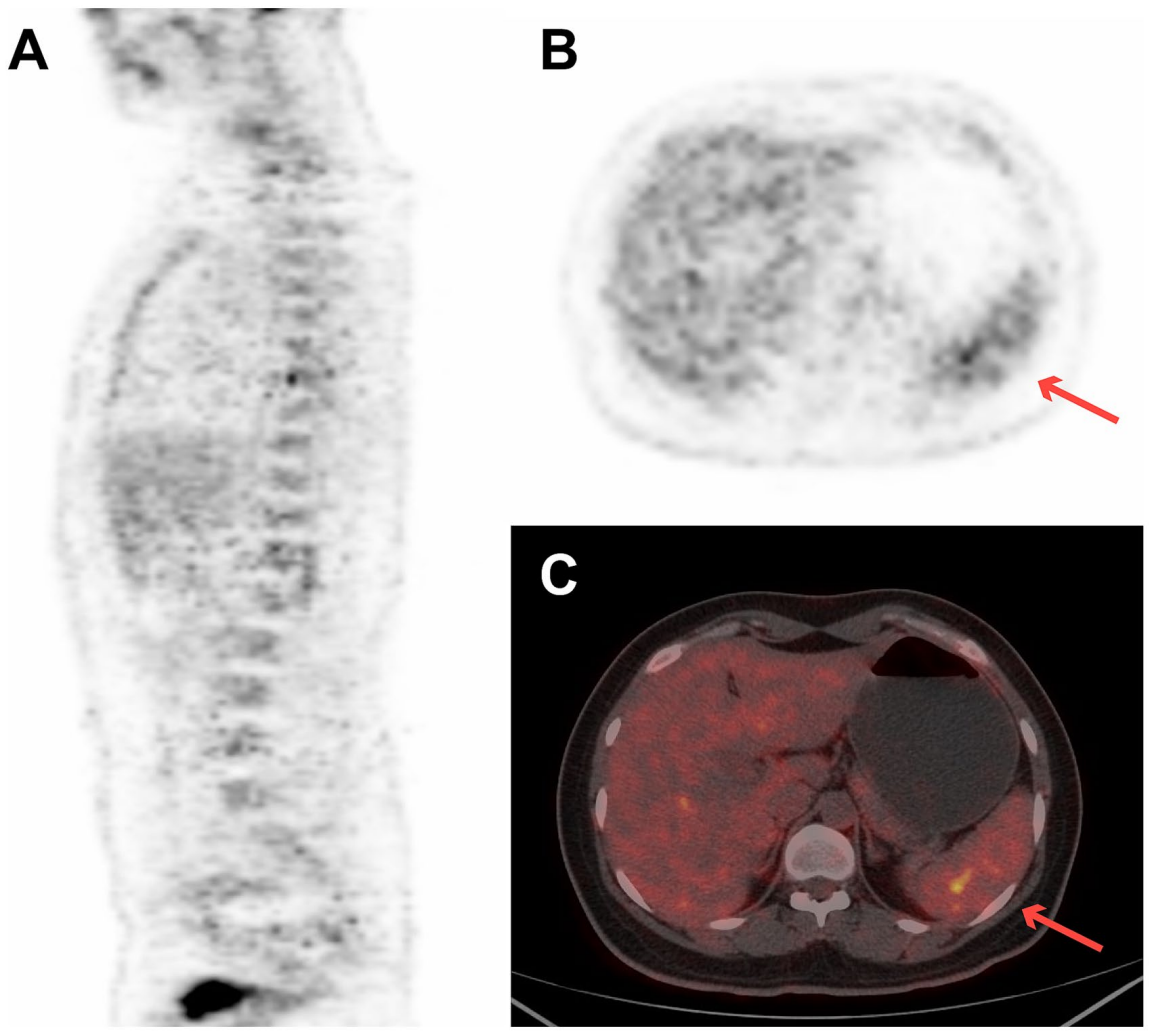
^18^F-FDG PET/CT images of case 5 revealed high FDG-avid of the spleen (SUVmax 4.4) and bones (SUVmax 9.1). (A) Sagittal PET image of bone, (B) transverse PET image of spleen (red arrow), and (C) transverse fused PET/CT image of spleen (red arrow).
